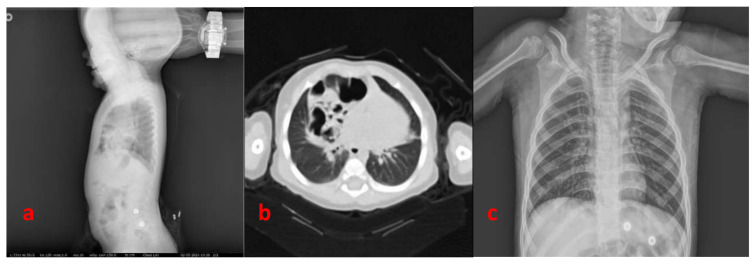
a) Lateral chest X-ray of a case of Morgagni hernia; b) chest tomography of the same case of Morgagni hernia; c) postoperative chest X-ray with the hernia reduced.
